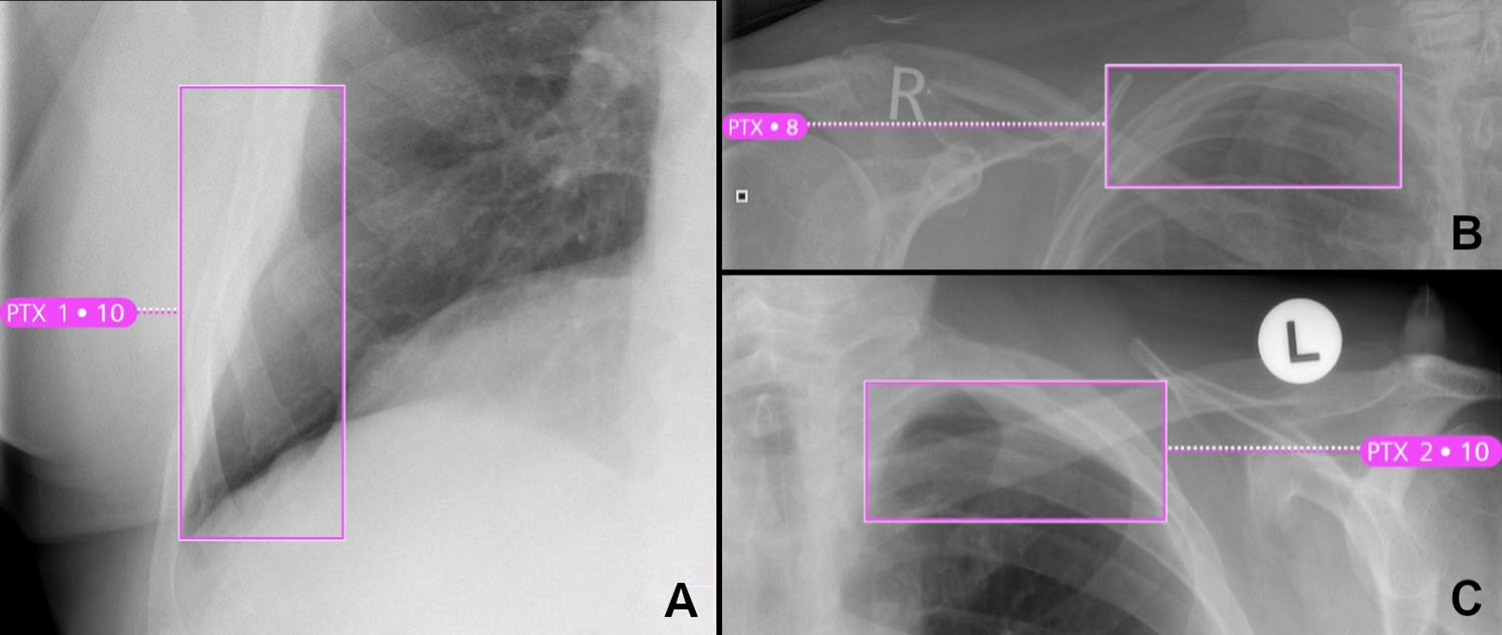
Three examples of false positively indicated pneumothoraces (A-C) by the AI Rad Companion Chest X-ray.
